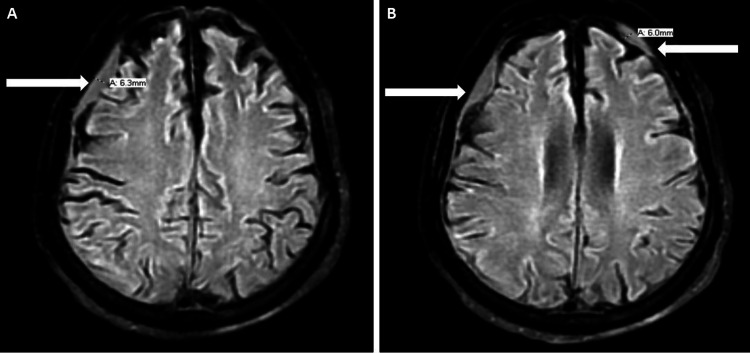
MRI brain T2-weighted axial flair (fluid-attenuated inversion recovery) sequence Figure 2A demonstrates a 6.3-mm rim of subacute subdural hematoma abutting the right frontoparietal lobe (white arrow). Figure 1B shows subtle rims of small, subacute subdural hematomas abutting the bilateral frontal lobes (white arrows).
